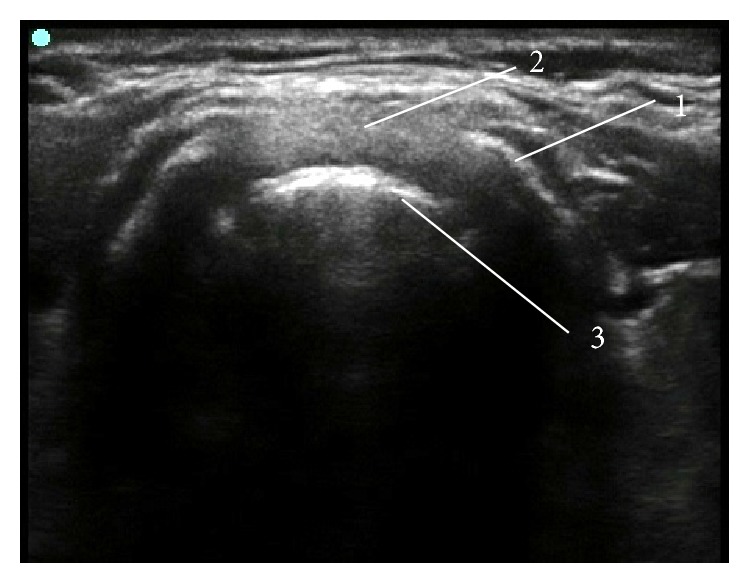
Ultrasound visualization of a stent inserted into the tracheal stenosis. (1) Tracheal wall, (2) intratracheal stenotic tissue, and (3) anterior part of the tracheal stent.
